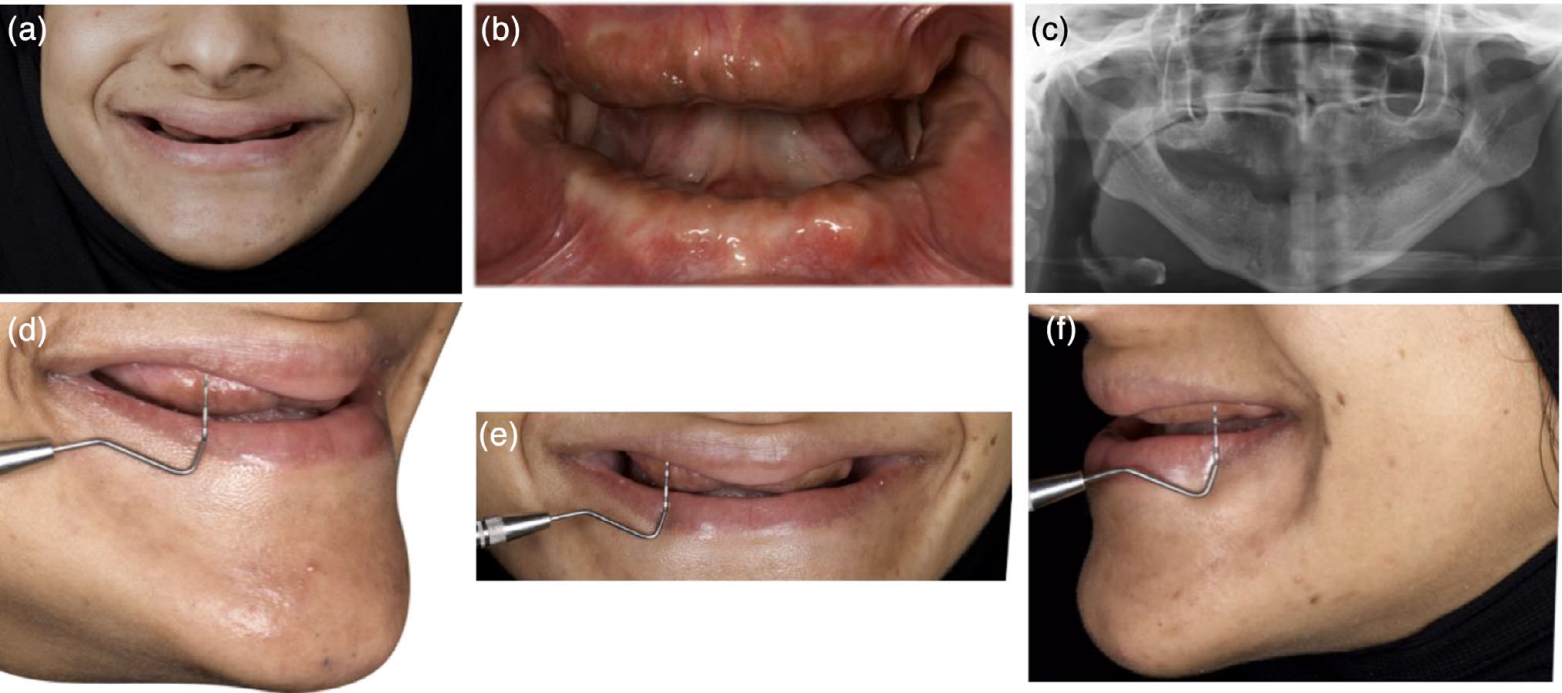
(a) Preoperative extraoral photograph; (b) intraoral photographs; and (c) orthopantomogram showing the edentulous maxilla and mandible. Extraoral photographs showing excessive bone display with nonuniform bone shape: (d) right profile view, (e) frontal view, and (f) left profile view.
